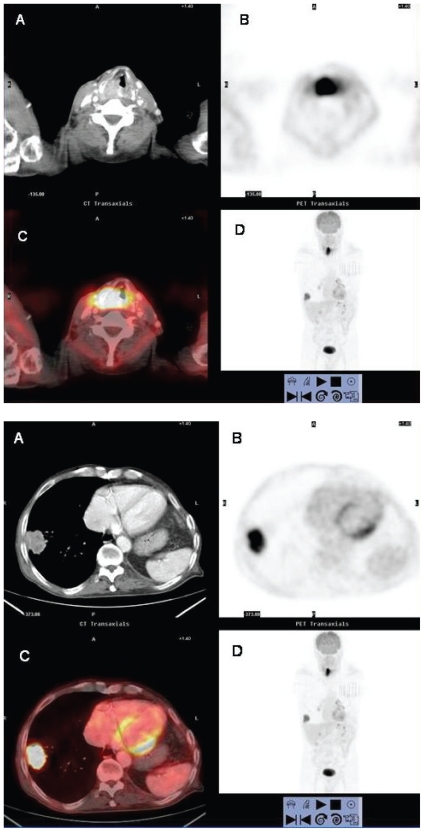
Synchronous lung cancer in a patient undergoing initial staging for laryngeal squamous carcinoma. 10A: axial contrast-enhanced CT (A), axial PET (B) and fused axial ^18^FDG PET/CT (C) images at the level of vocal cords show a mass with intense ^18^FDG uptake in the larynx consistent with laryngeal carcinoma. A focus of increased tracer activity is also identified in the right lung at the coronal PET scan (D). 10B: axial contrast-enhanced CT (A), PET (B), and fused ^18^FDG PET/CT (C) images at the lung bases. A peripheral lung mass abutting the pleura with a rim of increased ^18^FDG uptake is observed in the right lower lobe.
